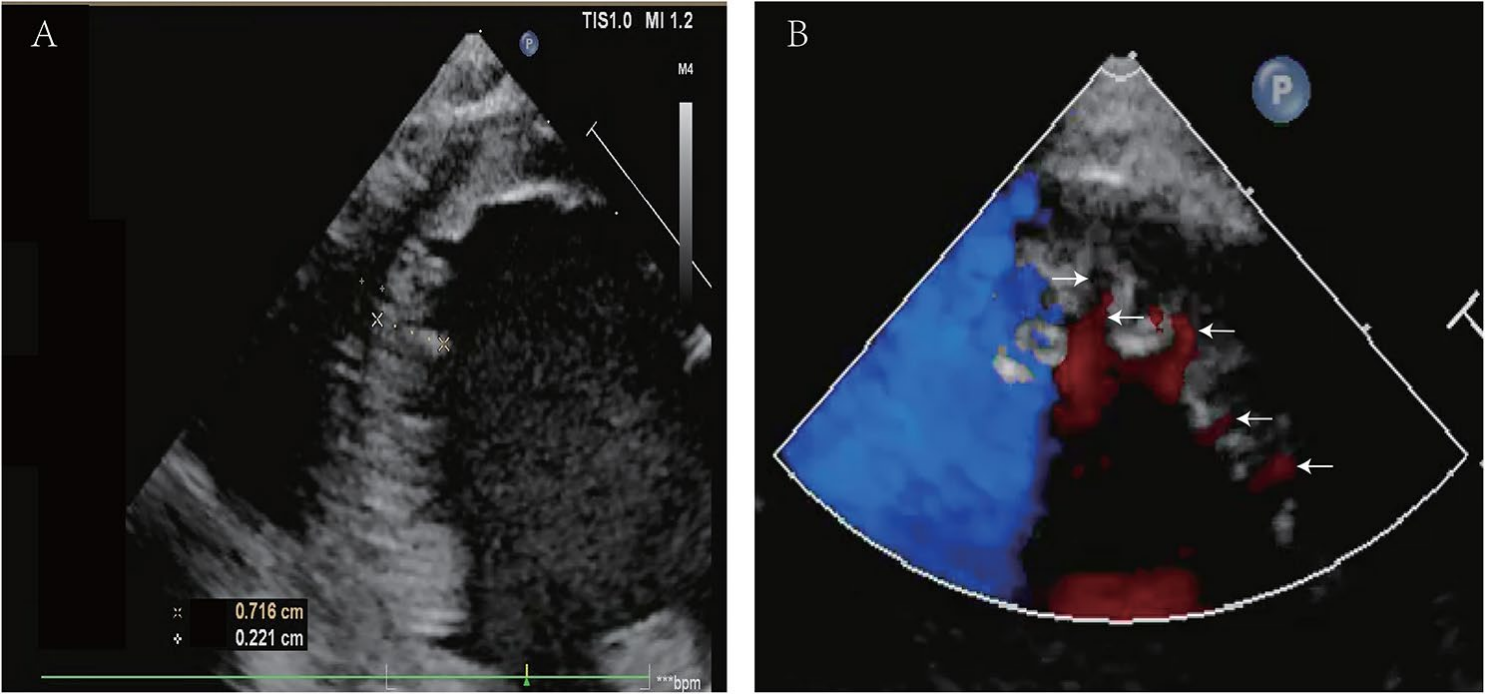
The ultrasound of the patient at 15 month old indicated noncompaction of ventricular myocardium. A: the noncompaction myocardium (× ×) was 0.716 cm and the compaction myocardium (+ +)was 0.221 cm (N/C = 3.27). B: Color doppler imaging showed deep intertrabecular recesses (→) and the appearance of blood flow from the ventricular cavity into the intertrabecular recesses (←)
